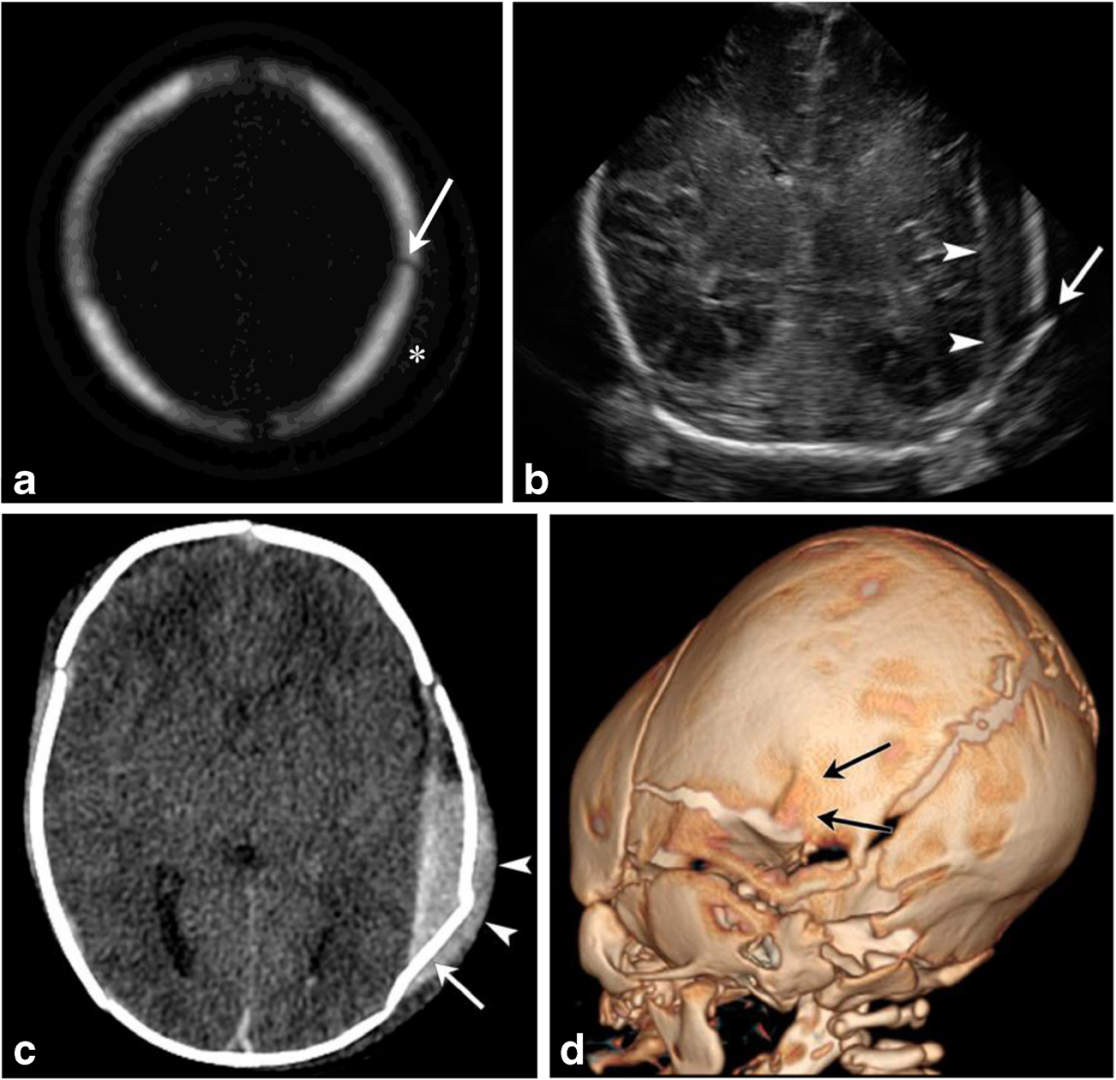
Skull fractures in two neonates Axial bony algorithm reconstruction from a head CT (a) in a 2-day-old demonstrates a non-displaced linear left parietal skull fracture (arrow) with overlying soft tissue swelling (marked by asterisk on a). Ultrasound and CT images on another 1-day-old male (b, c) with a history of traumatic delivery characterized by multiple attempts of vacuum extraction. Coronal gray scale ultrasound image (b) demonstrates a displaced left parietal fracture (arrow) with underlying extra-axial fluid collection (arrowheads). Axial non-contrast head CT image (c) shows a complex left parietal bone fracture with an angulated anterior component and an adjacent depressed “ping-pong” fracture component posteriorly (arrow). There is an associated overlying hyperdense fluid collection consistent with cephalhematoma (arrowhead). There is also an underlying large epidural hemorrhage with fluid/fluid levels. 3-D volume rendered image (d) re-demonstrates the complex left parietal bone fracture (black arrows)
